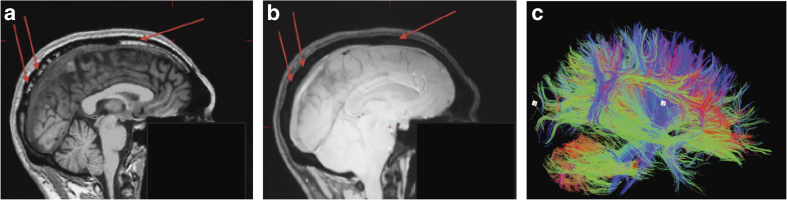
Example MRI data. (a) shows a sagittal section through T1-weighted image of one participant from the MPRAGE sequence (face removed), while (b) shows the same section through same participant using a contrast optimised for skull-brain contrast from the ME-FLASH sequence. The red arrows indicate tissue that makes it difficult to extract the inner skull/CSF boundary in the MPRAGE image but not the ME-FLASH image. (c) shows an example of the major white-matter tracts that can extracted from the Diffusion-Weighted data from the same participant.
